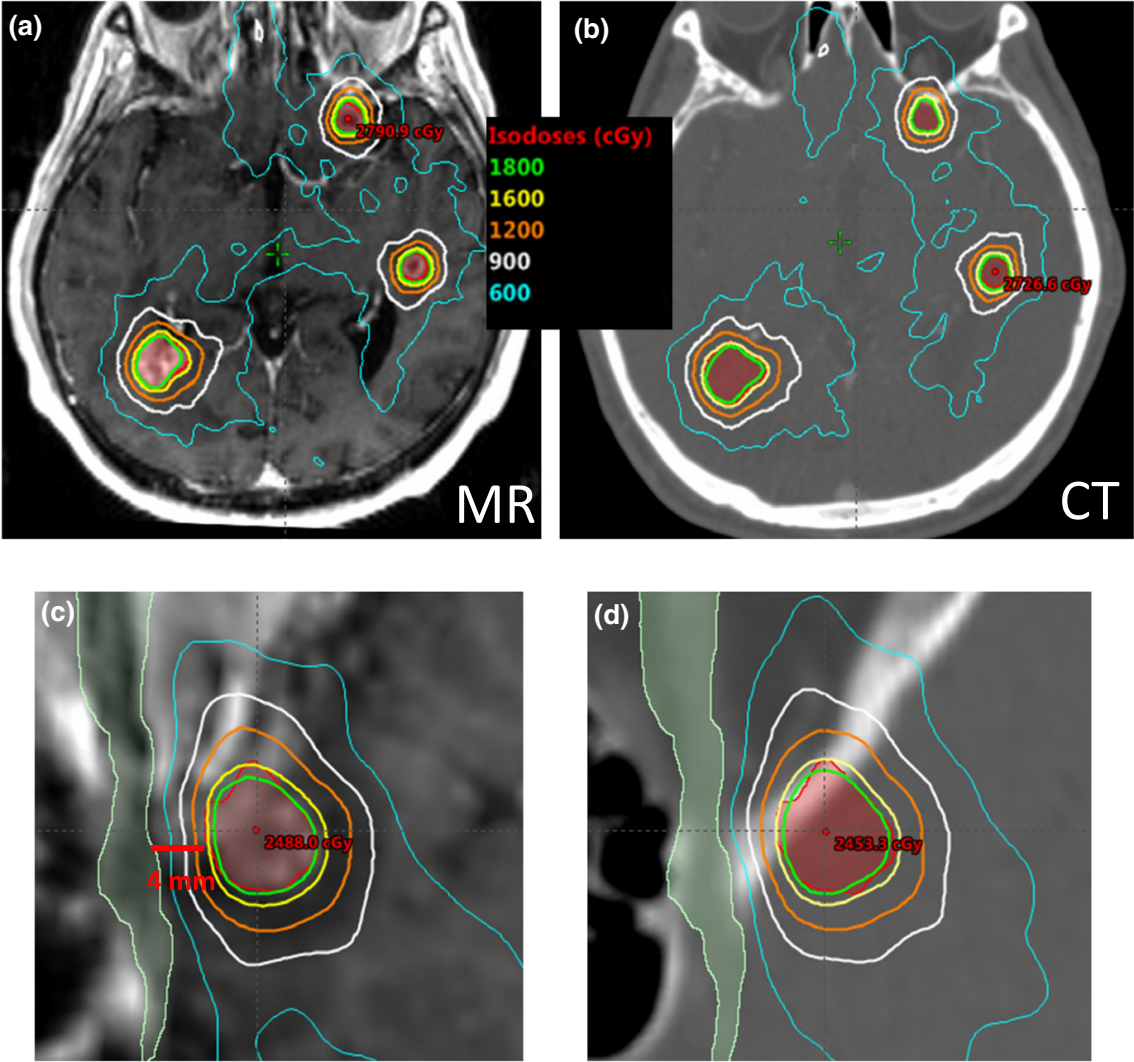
Dose distribution comparison of MR preplan and CT plan. The shape of isodose lines of the MR preplan in (a) and (c) were closely matched to those of the CT plan in (b) and (d). Preplanning enables thorough exploration of optimization performance, and can be of particular help to handle patient‐specific challenges ahead of the frame placement. Bottom images ((c) and (d)) show the close‐up dose distributions for a target volume (red) near the optic nerve (light green)
